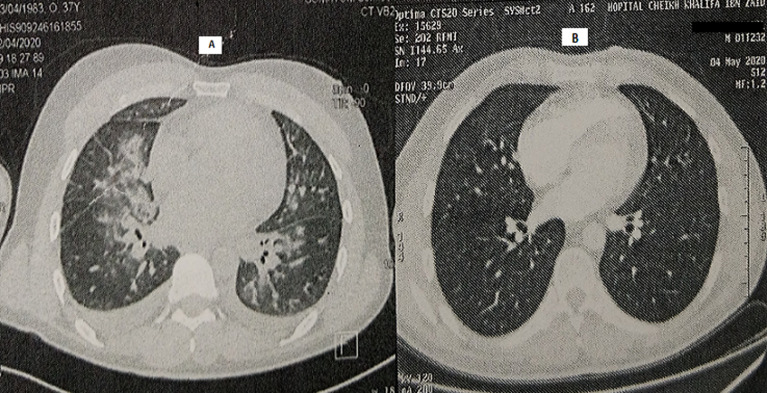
chest computed tomography: (A) obtained on day 1 shows substantial hilar congestion, cardiomegaly, and ground-glass changes; (B) obtained on discharge shows no significant abnormality
